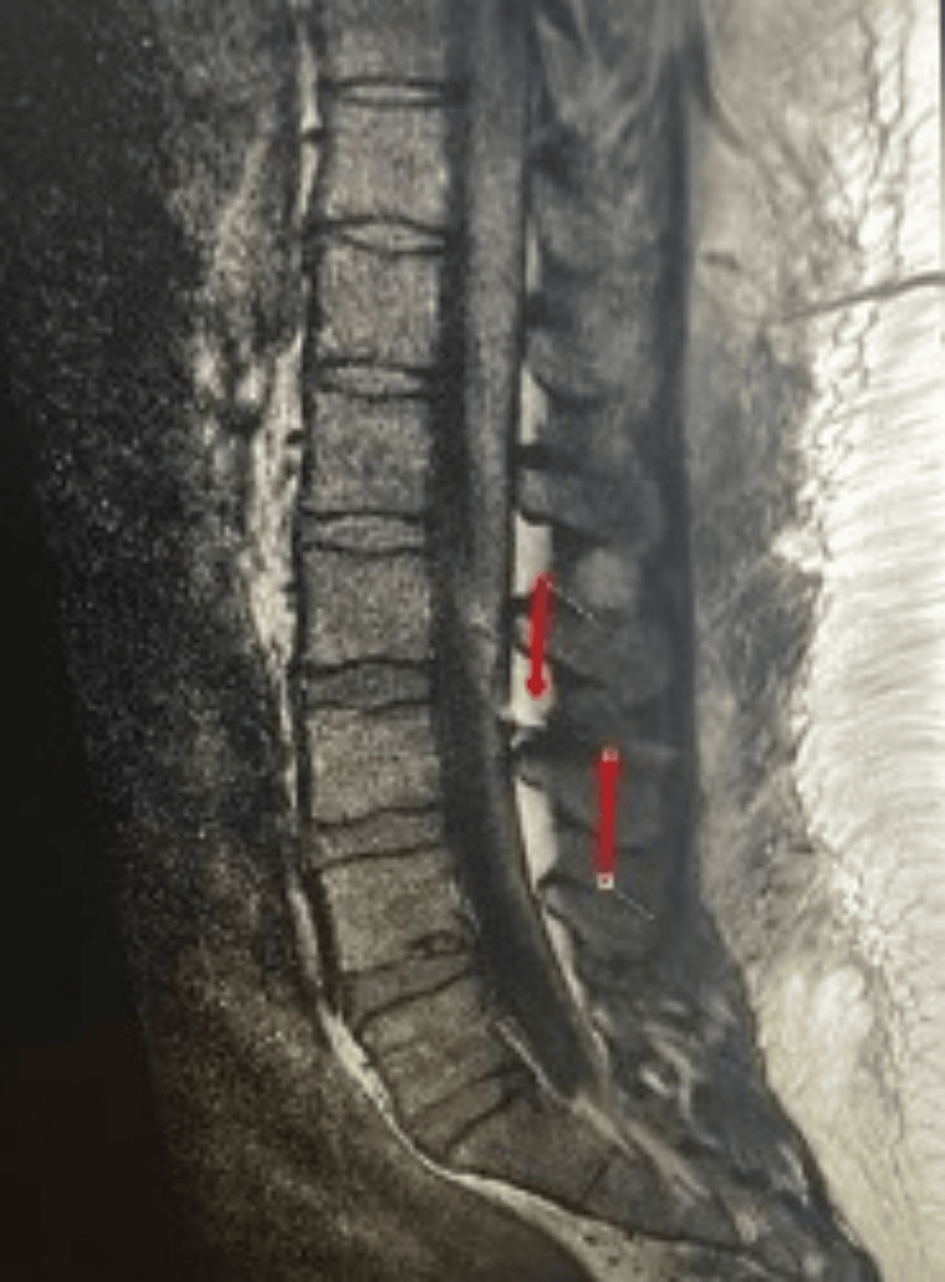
MRI of the spine. The epidural catheter is seen at the L2-L3 level, with its looped intrathecal component. The tip likely ends at the left L2-L3 foramina.
